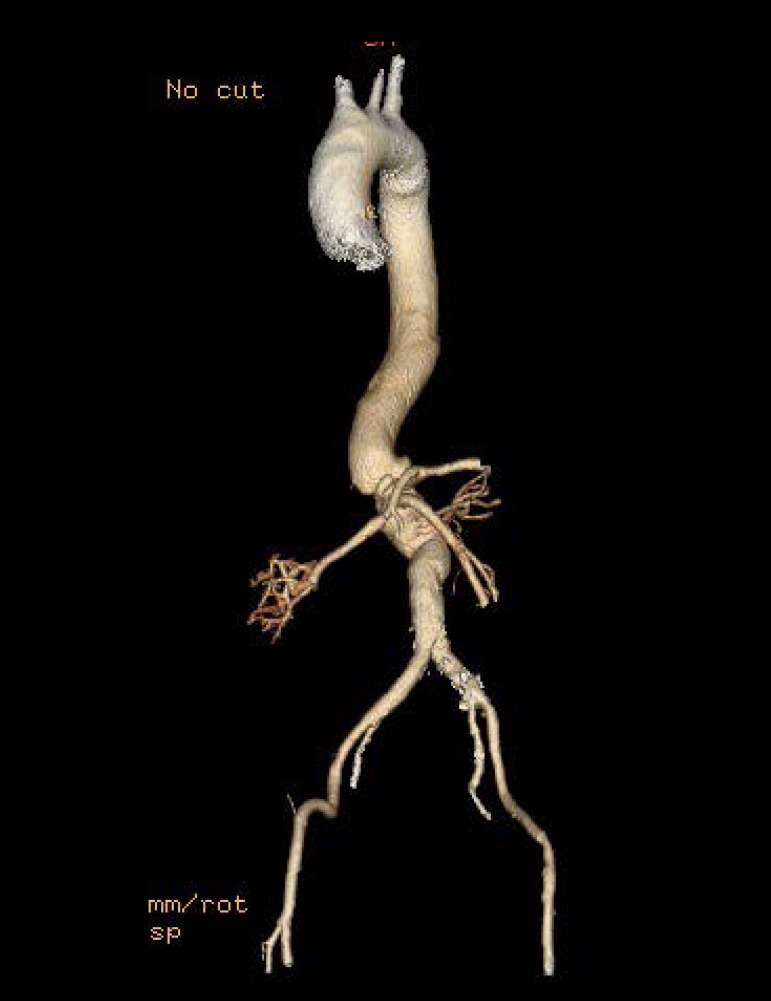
Postoperative CT angiography demonstrating a good result after open
